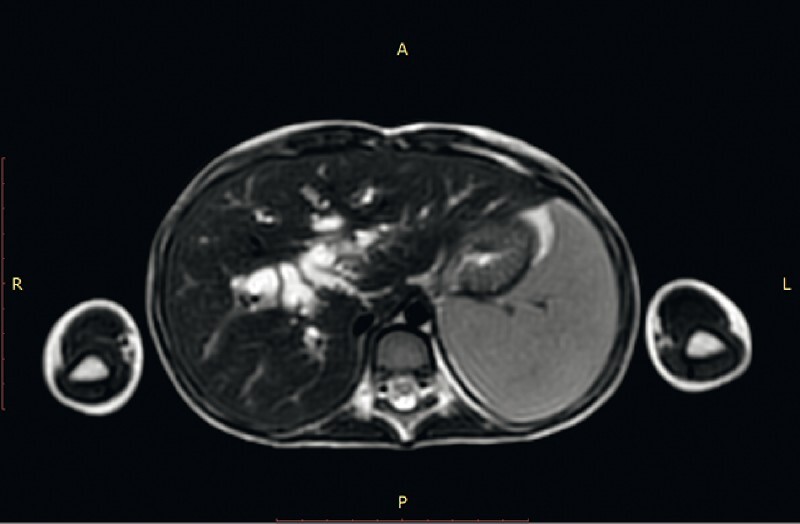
Abdominal magnetic resonance imaging. Dilation of intra- and extrahepatic bile ducts, and possible parasitic structures in the common bile duct, cystic duct, and gallbladder lumen. Patchy, subtle T2 signal enhancement near the bile ducts in the liver suggestive of cholangitis.
